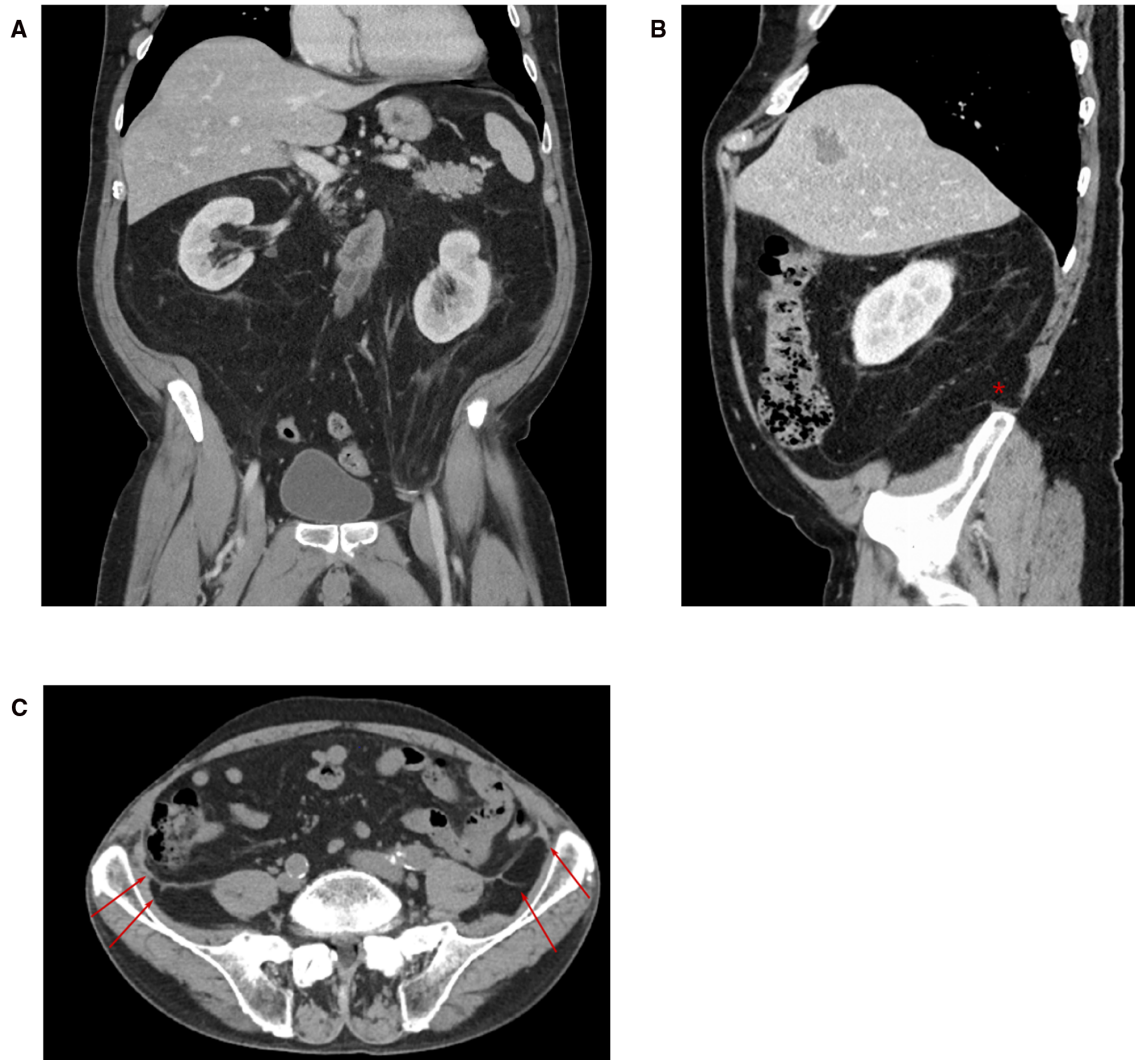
CT imaging with coronal (A) and sagittal (B) sections through the abdomen. The pararenal fat extends into the pelvis and in this patient ultimately into the left inguinal canal like an inverted cone. An attachment of the posterior renal fascia to the transversalis fascia at the ililac crest can be seen in the sagittal section (*) and (C) transverse section (arrows).
